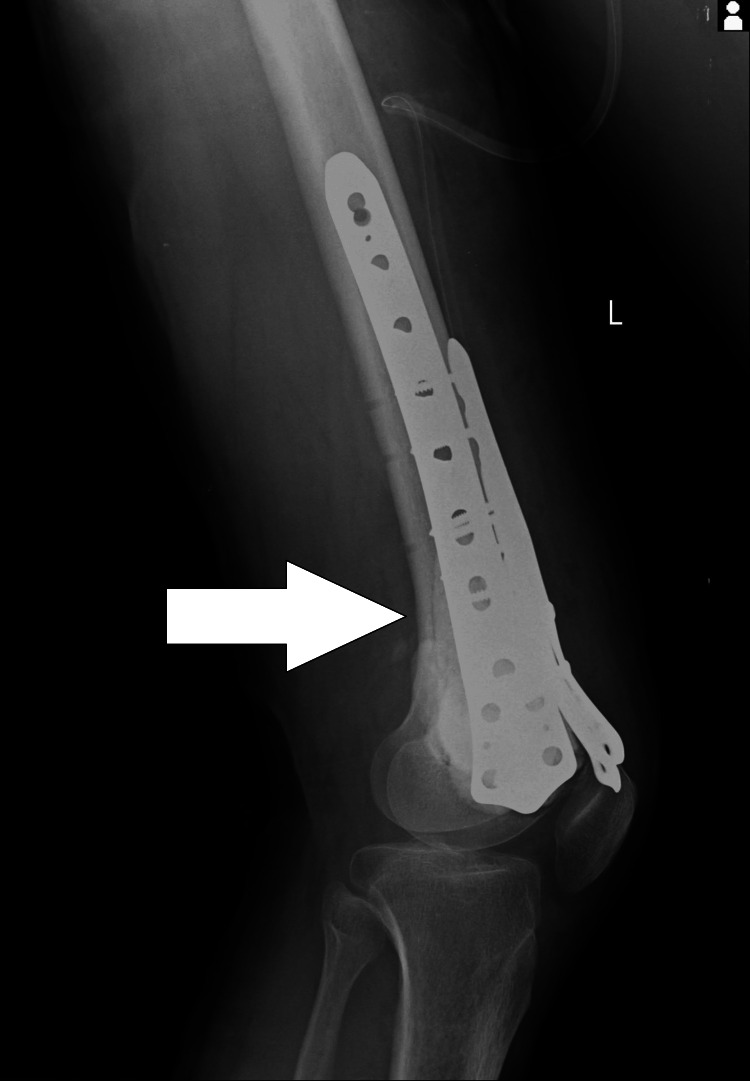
X-ray image showing lateral view post operative
